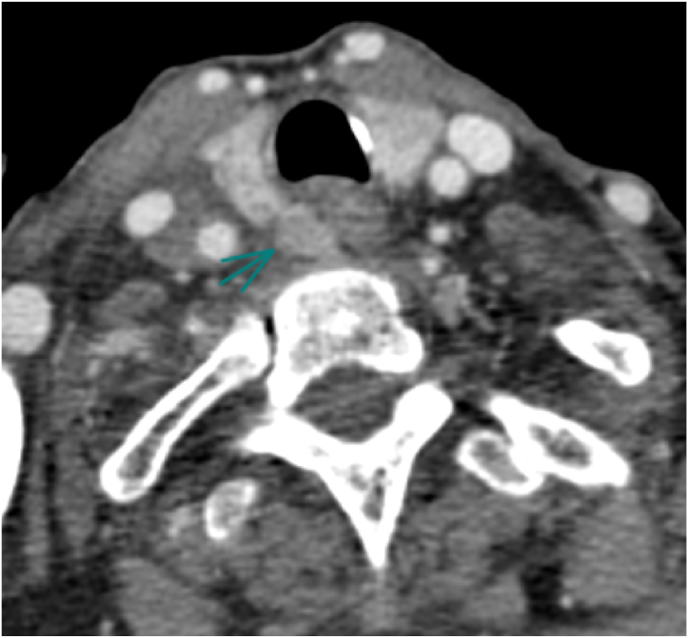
cervical CT scan reveling the adenoma with no surgical indication.
